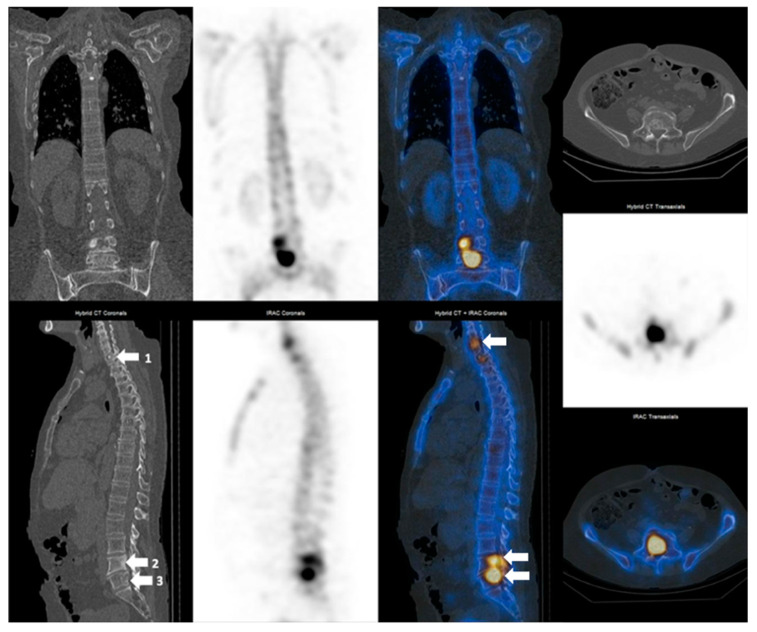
69 year-old breast cancer female patient with grade 2 invasive ductal carcinoma. First SPECT-CT scanning revealing both osteolytic and osteoblastic metabolically active metastatic lesions (1—degenerative lesion SUVmax 24.1 g/mL T1 vertebra, 2—osteoblastic lesion SUVmax 27.7 g/mL L4 vertebra, 3—osteolytic lesion SUVmax 76.9 g/mL L5 vertebra).
